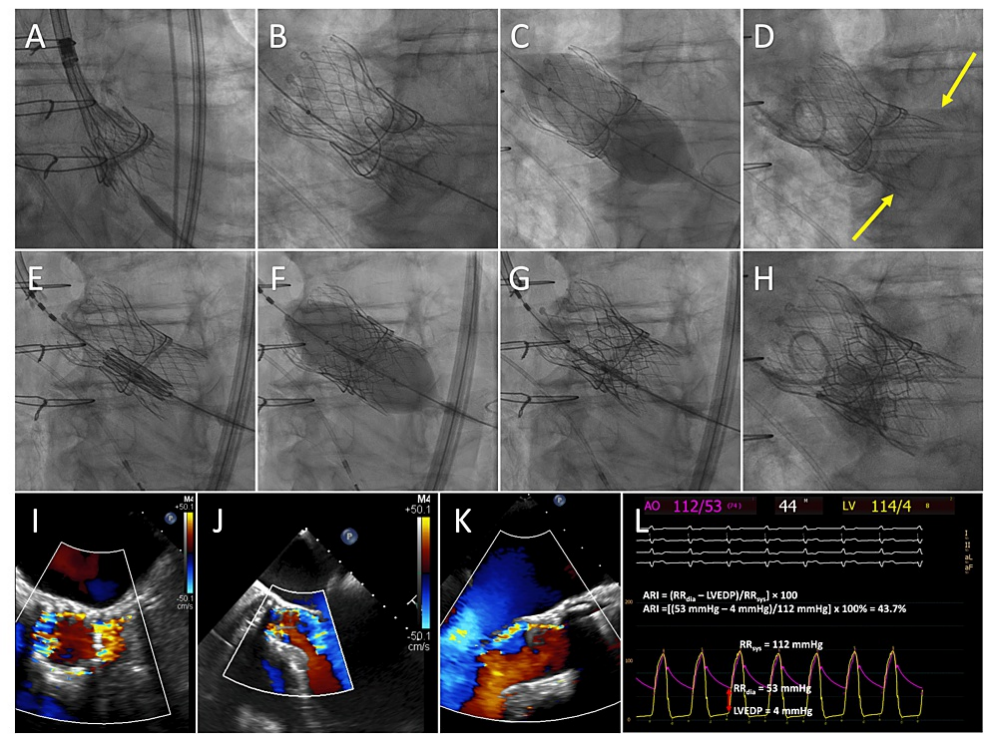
Intraprocedural TEE and fluoroscopic guidance for planned TAV-in-SAV with salvage S3-in-Evolut for treatment of severe PVL. (A) The prosthesis during the last phase of valve deployment, immediately before it is fully released. The implantation depth is measured from the hypothetical aortic annular plane to the interventricular end of the THV. Distance evaluations are made in relation to both the LCC and the NCC. (B) Micro-dislodgement of the THV occurred in the direction of the LVOT by 3 mm in relation to the LCC and 3 mm to the NCC, respectively. (C) The Magna Ease valve was cracked with a 26 mm non-compliant balloon. (D) LVOT migration up to 17 mm of the Evolut occurred after BVF with consequent acute severe PVL (yellow arrows). (I-K) TEE revealed severe supra-skirt PVL. (E) A 26 mm S3 valve was positioned with the marker on the Magna Ease stent border (F and G) and deployed, (H) successfully sealing the PVL with only trace residual PVL (L) and favorable transvalvular hemodynamics. TEE: transesophageal echocardiography; TAV-in-SAV: transcatheter aortic valve in surgical aortic valve; S3: SAPIEN 3 balloon expandable valve; Evolut: Evolut R self-expanding valve; PVL: perivalvular leak; THV: transcatheter heart valve; LCC: left coronary cusp; NCC: non-coronary cusp; LVOT: left ventricular outflow tract; BVF: bioprosthetic valve fracture; LVEDP: left-ventricular end-diastolic blood pressure; RRdia: end-diastolic blood pressure in the aorta; RRsys: systolic blood pressure in the aorta
